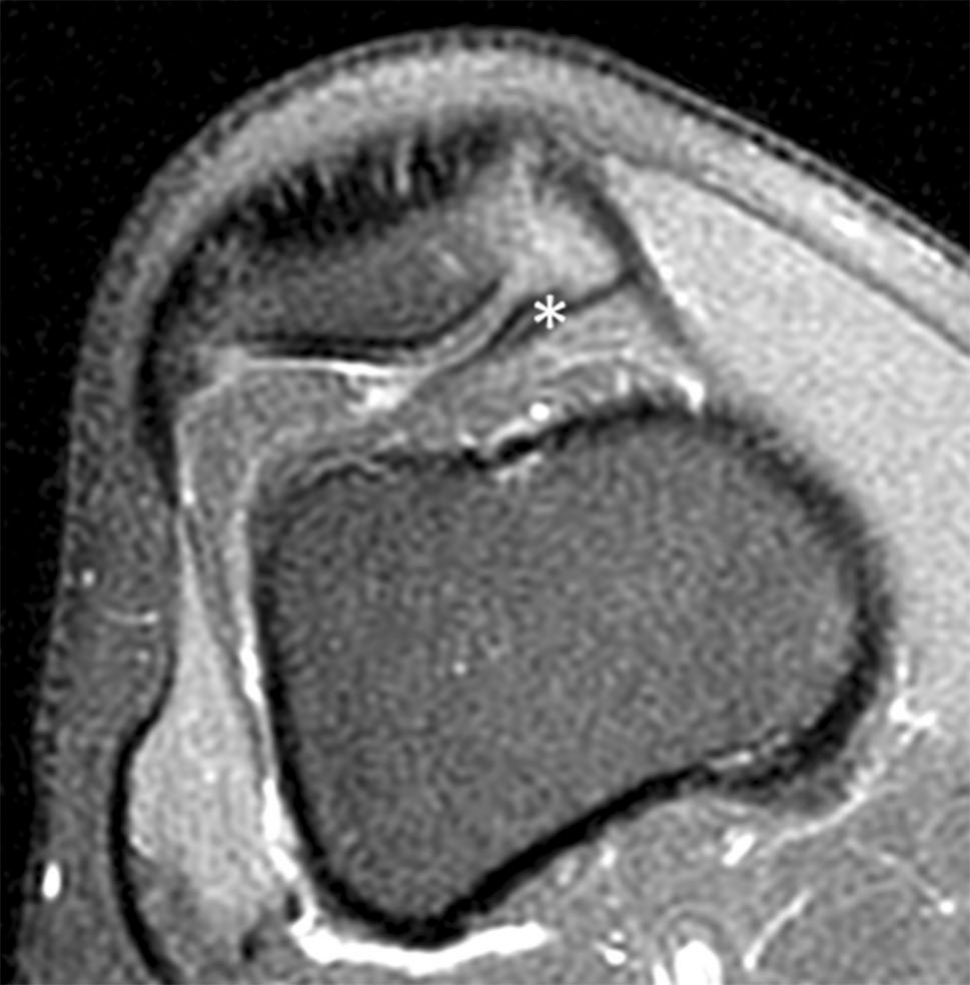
Magnetic resonance imaging of a duplicated medial plica (asterisk)
